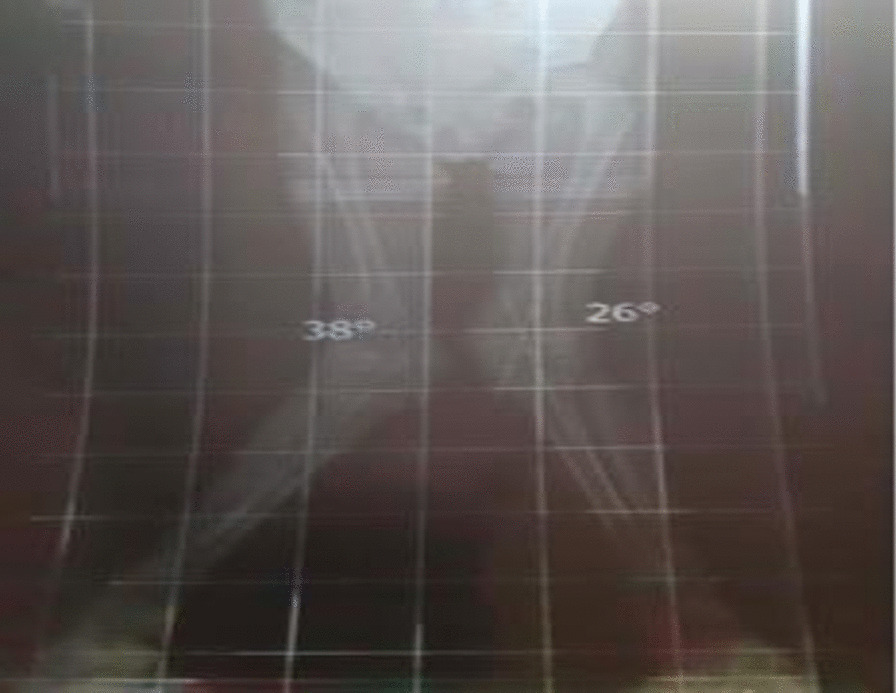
Bilateral lower extremity x-ray showing deterioration of the genu valgum deformity and decreased bone density. Right fibular shaft fissure fracture line without displacement noted with attempts at healing. Normal articulation of joint spaces
